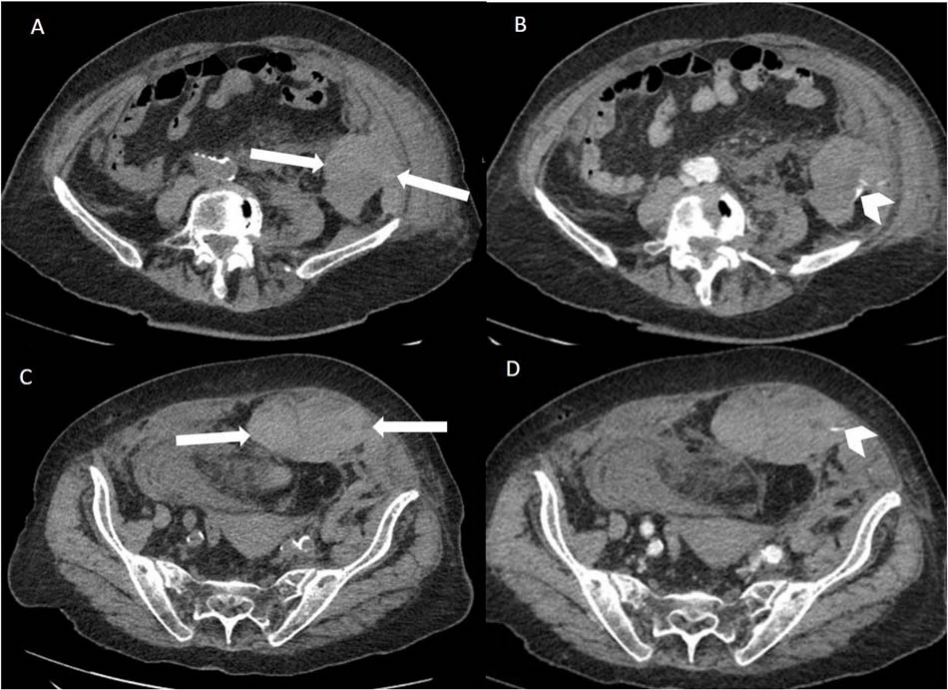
Axial non-contrast and post-contrast (100-s delay) CT abdomen and pelvis slices demonstrating two further left abdominal wall and pre-peritoneal collections (white arrows) with high attenuation on non-contrast slices (A and C) in keeping with haematoma. There is contrast blush in two locations (white chevrons in B and D) in keeping with active bleeding. The vascular territories that have likely contributed to bleeding include branches of left inferior epigastric and left lumbar arteries.
